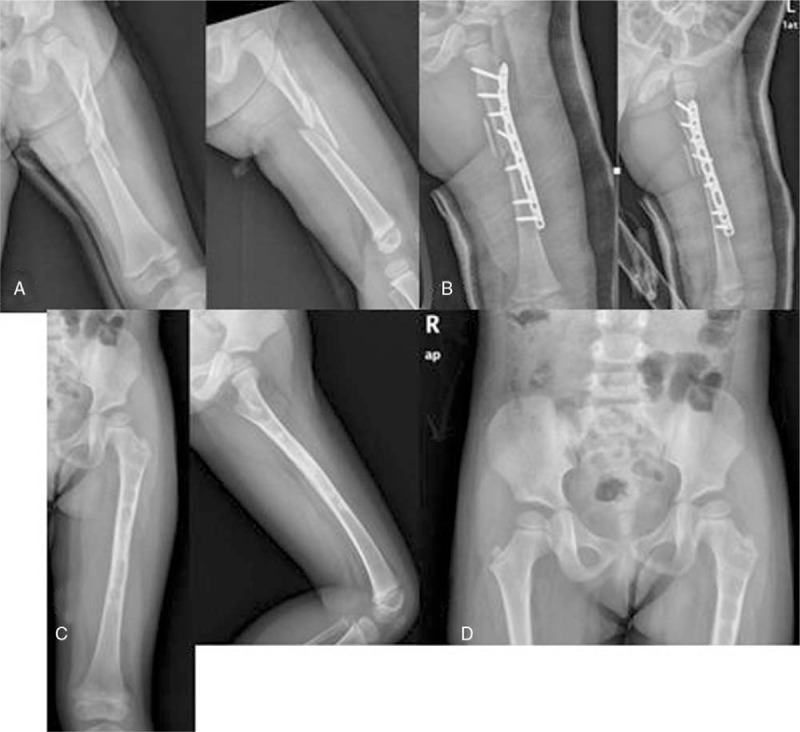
A 5.5-year-old boy suffered traffic accident. (A) AP and lateral radiographs of the right femur with comminuted unstable fracture of thesubtrochanteric region to shaft. (B) The patient was treated by open reduction with locking compression plate fixation. (C) Ten months after surgery, the fracture showed good healing radiographically and internal fixation was removed. (D) AP view of pelvis showed a symmetric NSA and no limb length inequality.
